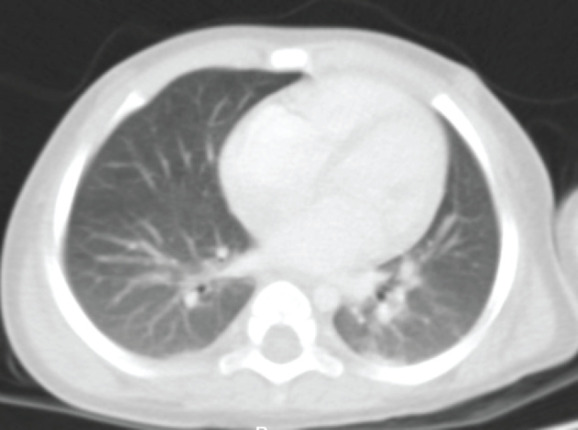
Axial contrasted chest CT scan. Smooth pleural surfaces are observed and there is no evidence of alterations. The pulmonary parenchyma shows dense irregular areas with scarce air bronchogram located in posterior segments of the apical regions with a diameter of less than 3 mm with no changes upon contrast application. The trachea, main bronchi and bronchovascular structures of the pulmonary hilar bronchi are normal. Source: Medical registry.
